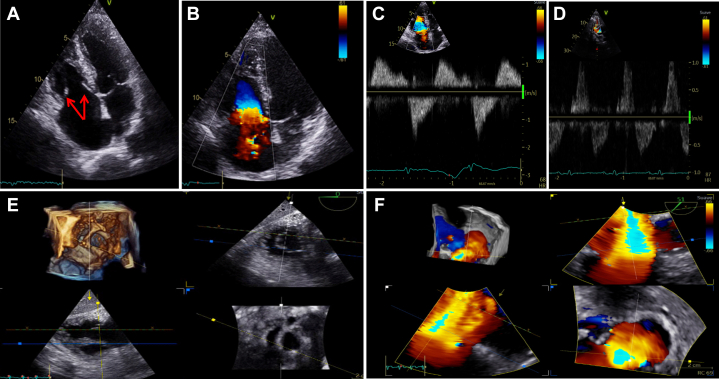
Preoperative Transthoracic and Transesophageal Echocardiography of the Tricuspid Valve (A) Transthoracic apical 4-chamber view. Red arrows indicate thickened and restricted motion of septal and mural tricuspid leaflets with a wide coaptation gap in systole. (B) Transthoracic apical 4-chamber view with color Doppler. Massive tricuspid regurgitation with a vena contracta of 14 mm. (C) Tricuspid valve continuous-wave Doppler showing no significant stenosis (mean pressure gradient 2.6 mm Hg; inflow velocity-time integral (VTI) 38 mL; pressure half-time 108 ms) and dense triangular regurgitation curve. (D) Hepatic vein flow showing systolic flow reversal. (E) Three-dimensional (3D) transesophageal echocardiographic reconstruction showing thickened and restricted motion of all tricuspid leaflets during systole. (F) 3D transesophageal reconstruction of tricuspid regurgitation. Planimetry revealed an effective regurgitant orifice area of 2.0 cm^2^ (torrential tricuspid regurgitation).
